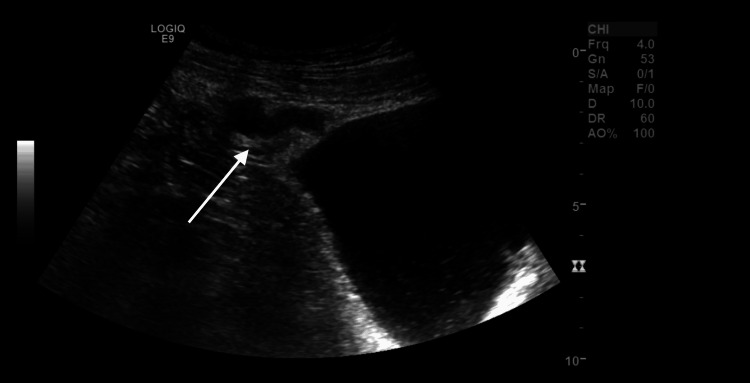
Ultrasound image demonstrating a tubular, cystic lesion at the bladder dome (arrow), consistent with a vesicourachal diverticulum.
